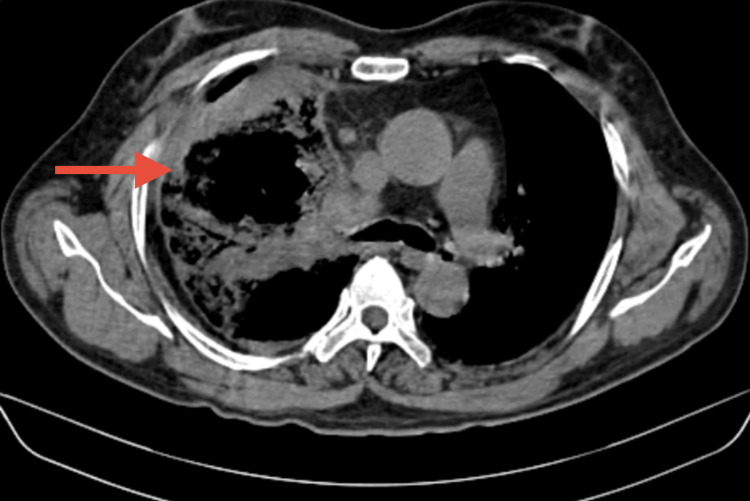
CT imaging of the thorax mediastinal place shows a cystic cavity with surrounding consolidation in the right upper and middle lobe.
